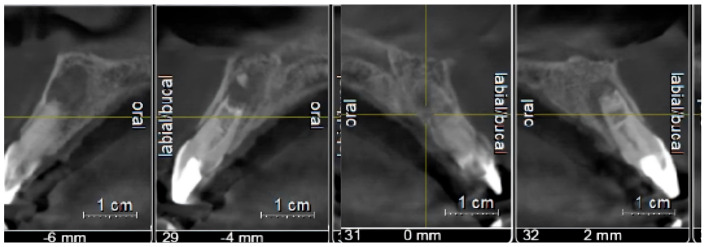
CBCT taken at 4-year follow-up. Axial section demonstrating the apical repair of teeth 11 and 21.
